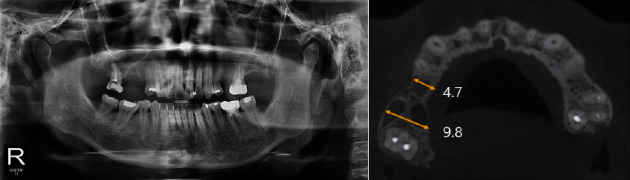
Pre-operative radiographic views (Case 3). In the CBCT view, it can be seen that the width and height of the alveolar bone are atrophied.
